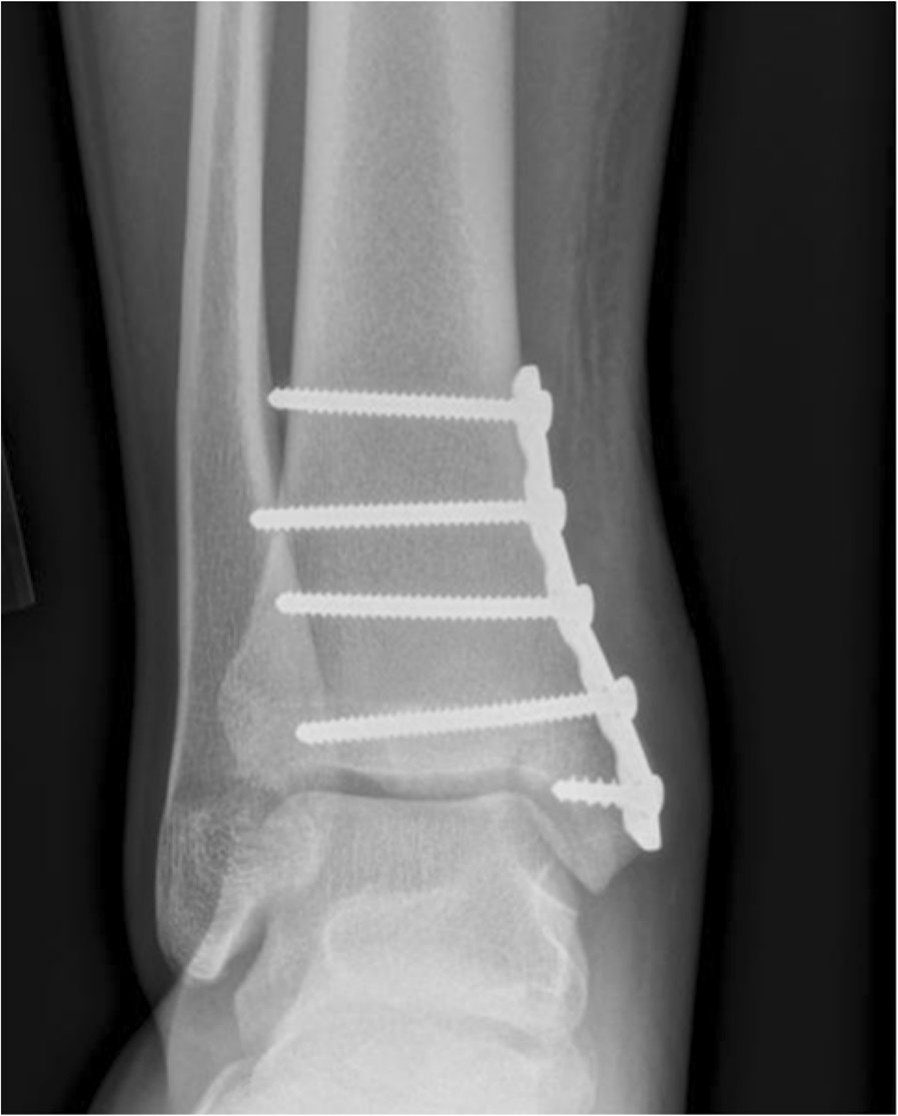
Post-fixation radiograph of a collegiate soccer player with medial malleolar stress fracture
